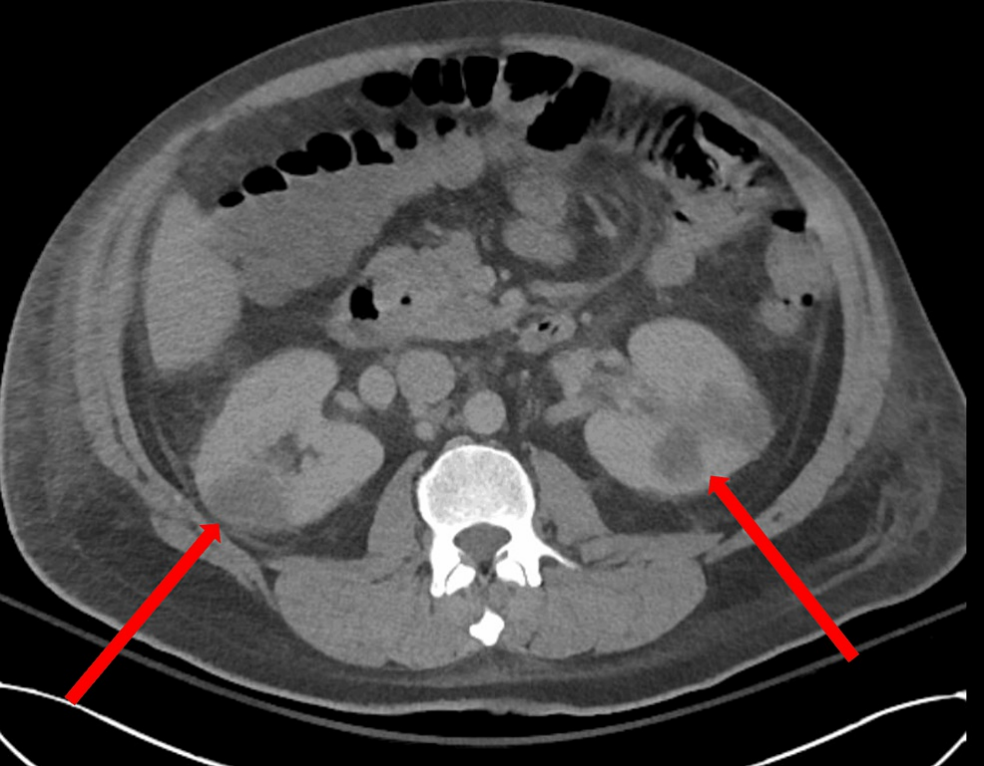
A non-contrast CT scan of the abdomen demonstrates bilateral renal cortical infarcts (arrows).
